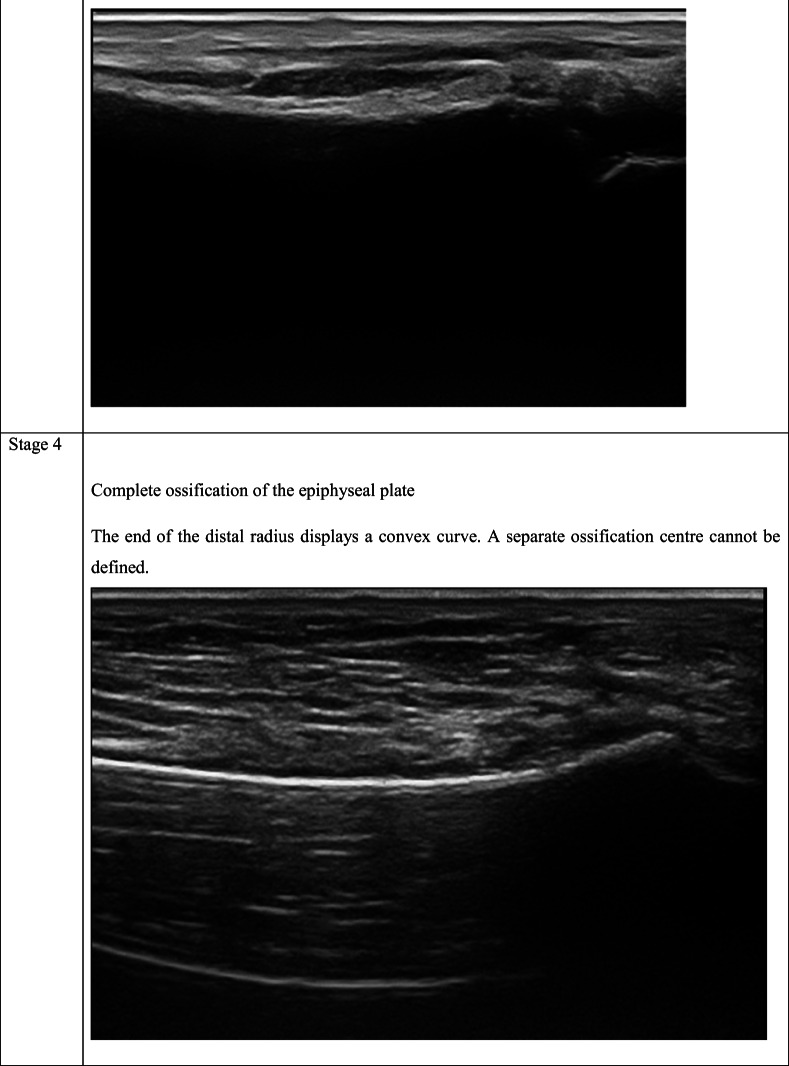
The ultrasonography staging system described by Schulz et al. [27] for the clavicle modified by Schmidt et al. [25] for the distal radius. Ultrasonographic images for each stage were shown in the table
